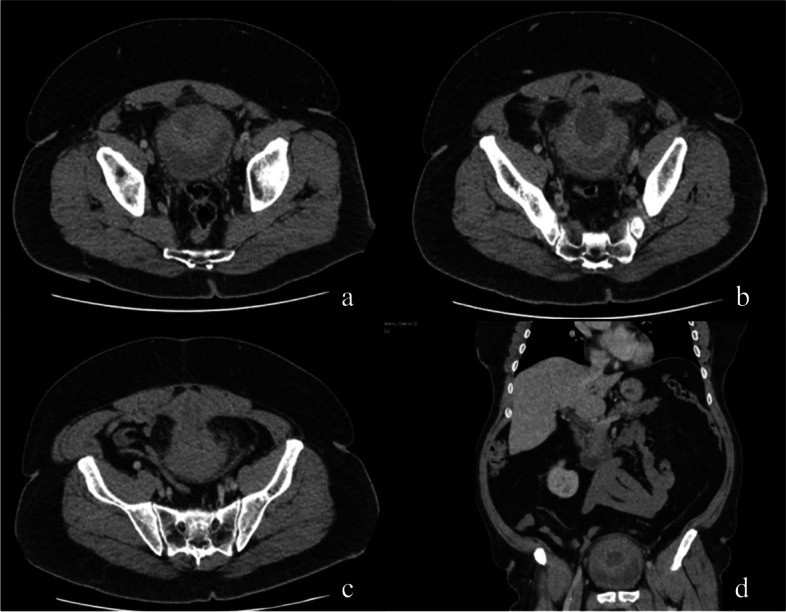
Pre-operative uninfused CT abdomen/pelvis showing a 10.3 cm solid and cystic mass abutting the superior bladder, extended up to the level of the umbilicus. a, b, c Cross-sectional, axial images showing the mass. d Coronal image showing the mass and omental nodularity
